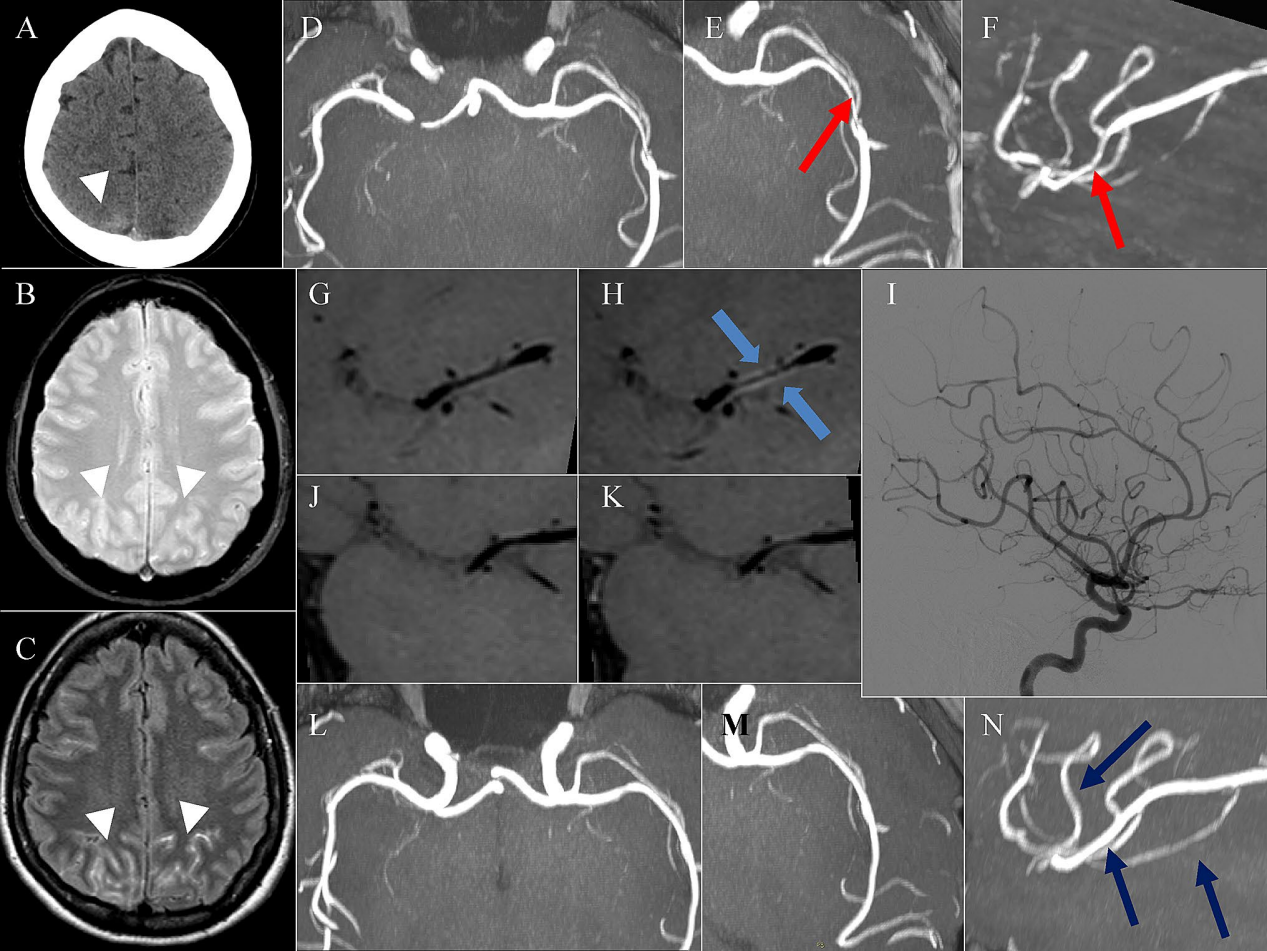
RCVS. A 56-year-old woman presented with an abrupt onset of headache and a CT scan demonstrated the presence of a small amount of subarachnoid hemorrhage in the right posterior parietal sulci (A). Nonvascular lesions responsible for the SAH were detected and thus the patient underwent brain MRI after few days, confirming the presence of a small amount of subarachnoid hemorrhage in the right posterior parietal sulci for the presence of hyperintensity in T2* and FLAIR sequences (B, C white arrowheads). The TOF sequence highlight the presence of caliber alterations in the left M2 segment (D–F, red arrows), and the Vessel Wall MRI study before (G) and after (H) the administration of contrast material demonstrated the presence of a slight circumferential enhancement in correspondence of the left M2 segment stenosis (blue arrows). Few days after the patient underwent a DSA angiography (I) that demonstrated the presence of a more diffuse caliber alterations in the intracranial arterial vasculature. The six months MRI follow-up demonstrates the complete resolution of the enhancement at Vessel Wall study before (J) and after (K) the contrast administration, as well as the caliber alterations (L–N blue arrows). The reversibility of those findings was then suggestive for a Reversible Cerebral Vasoconstriction Syndrome (RCVS), although non-enhancing concentric wall thickenings are more common than enhancing ones
